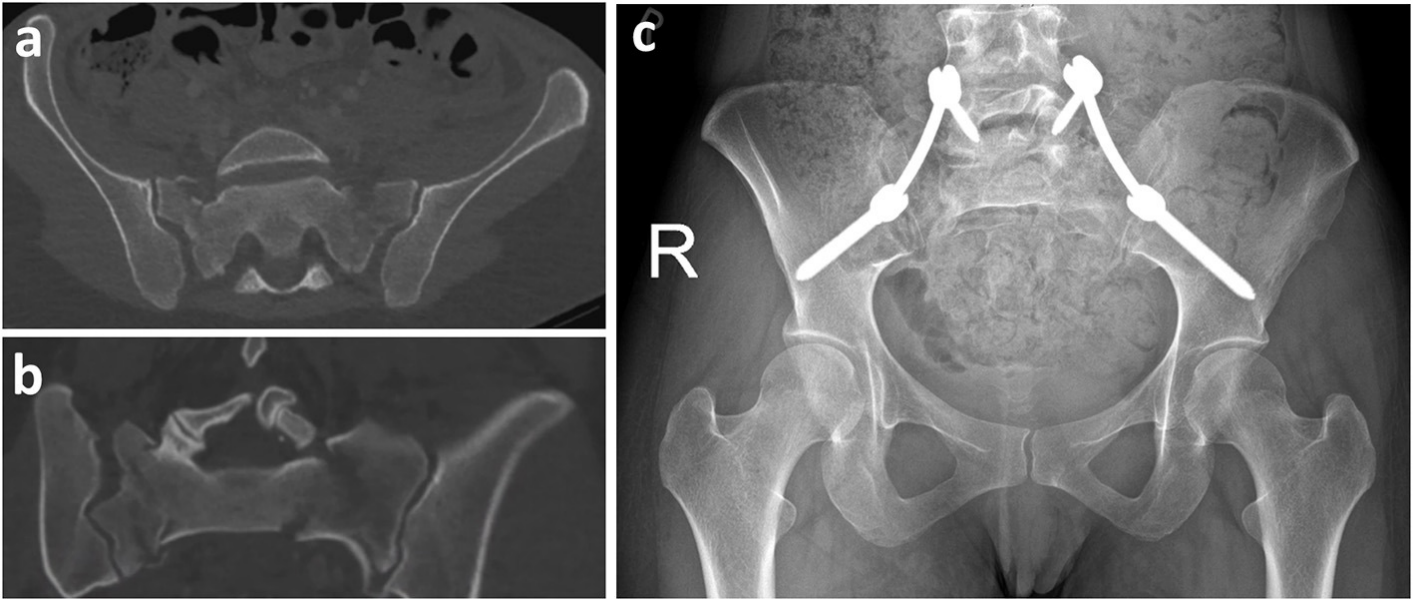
SPF. Female patient (29 years old) with spinopelvic dissociation and bilateral sacral fracture after road accident. The injury was classified (a: CT scan coronal, b: transversal) as AO C3.3. Surgery was performed with spinopelvic fixation (SPF) (L5 to Os ilium) (c).
